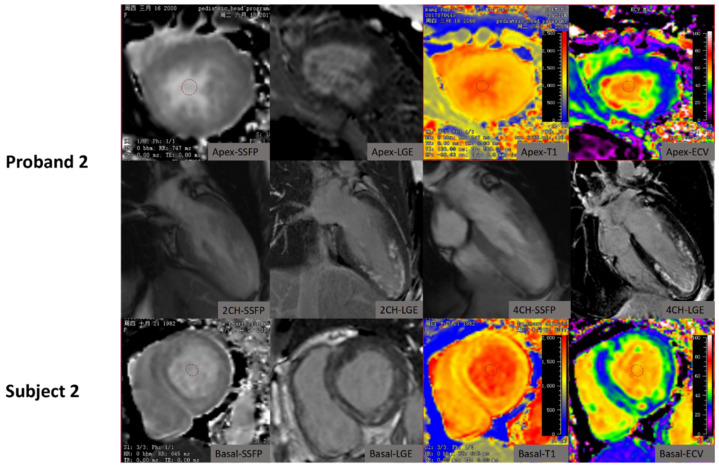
The characteristics of cardiac MRI in the proband 2 patient and the patient’s mother (Subject 2) with Danon disease.
